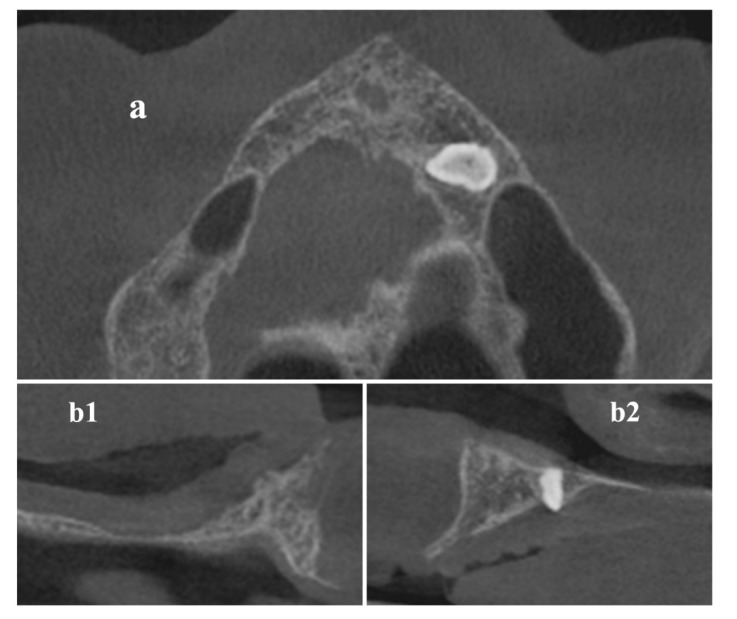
Cone-Beam-Computed-Tomography (CBCT) axial (a) and cross-sectional (b1,b2) images before surgical procedure.
